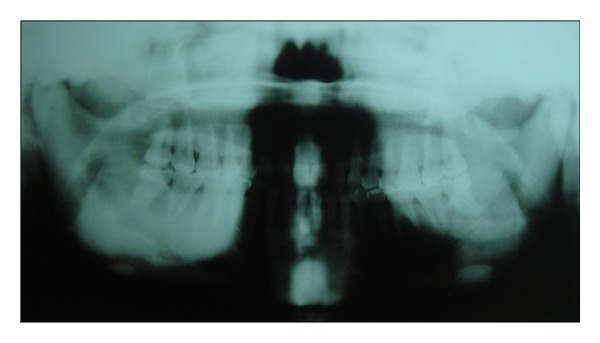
Panoramic radiograph showing signs of early periodontitis and sclerosis of the mandible and maxilla.
